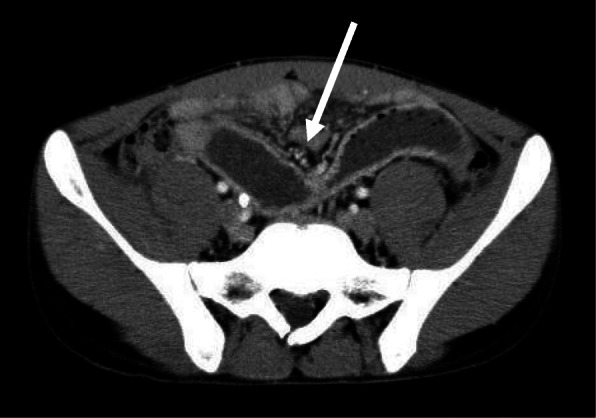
CT showing stenosis of the small bowel and dilatation of the oral small bowel
